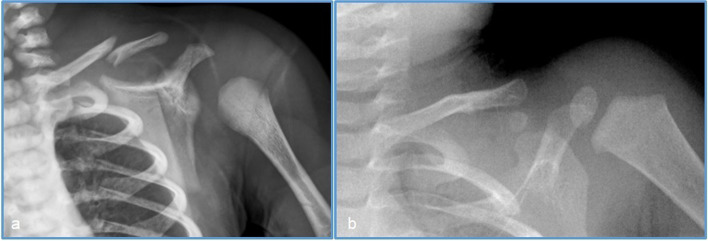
Male neonate, instrumented delivery, with a left clavicle fracture, diagnosed on the second day of life after perception of decreased mobility of the upper limb and crepitus of the clavicle. a X-ray on day 2 showing a middle third left clavicle fracture with deviation. b At 10 months follow-up: patient clinically without symptoms or restrictions and the X-ray shows clear evidence of consolidation and remodeling of the fracture
